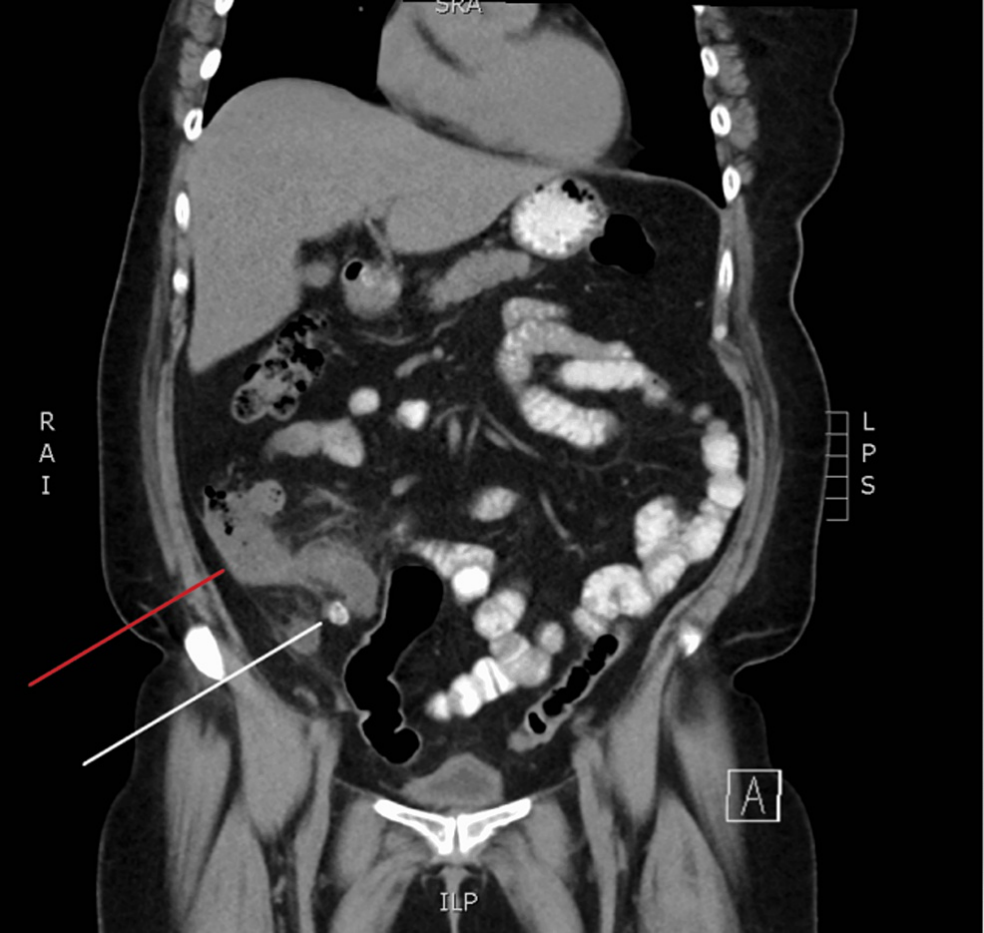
CT scan of the abdomen and pelvis with oral contrast (coronal slice). Red marker indicating cecal thickening. White marker indicating fecalith. CT: computed tomography
